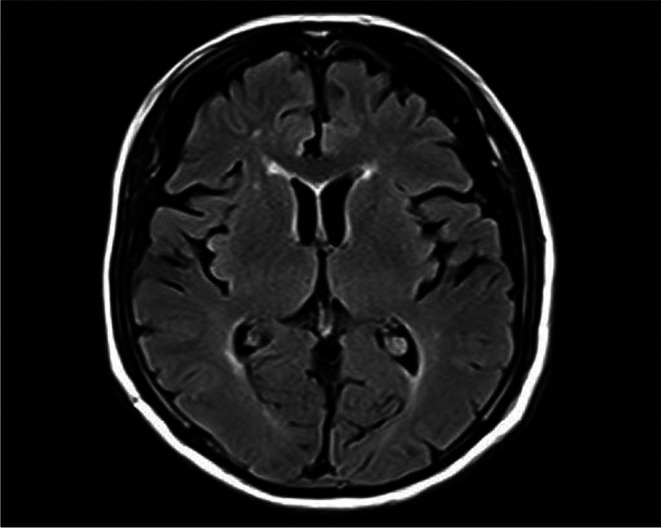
FLAIR‐weighted brain MRI on hospital day 1 showing no localized atrophy or abnormal signal intensity. No encephalitic changes were observed. FLAIR, Fluid‐attenuated inversion recovery; MRI, Magnetic resonance imaging.
